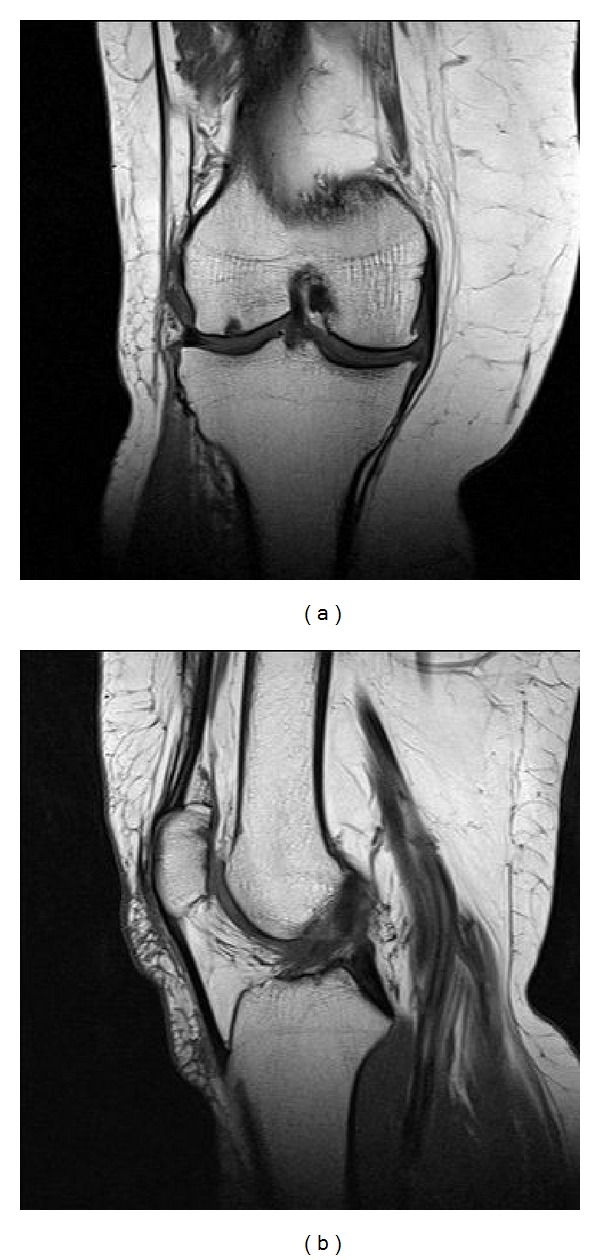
Preoperative T1-weighted MR image of the right knee, revealing complete tear of the medial meniscus, degeneration of the tibiofemoral joint cartilage, and chondromalacia of the patella.
